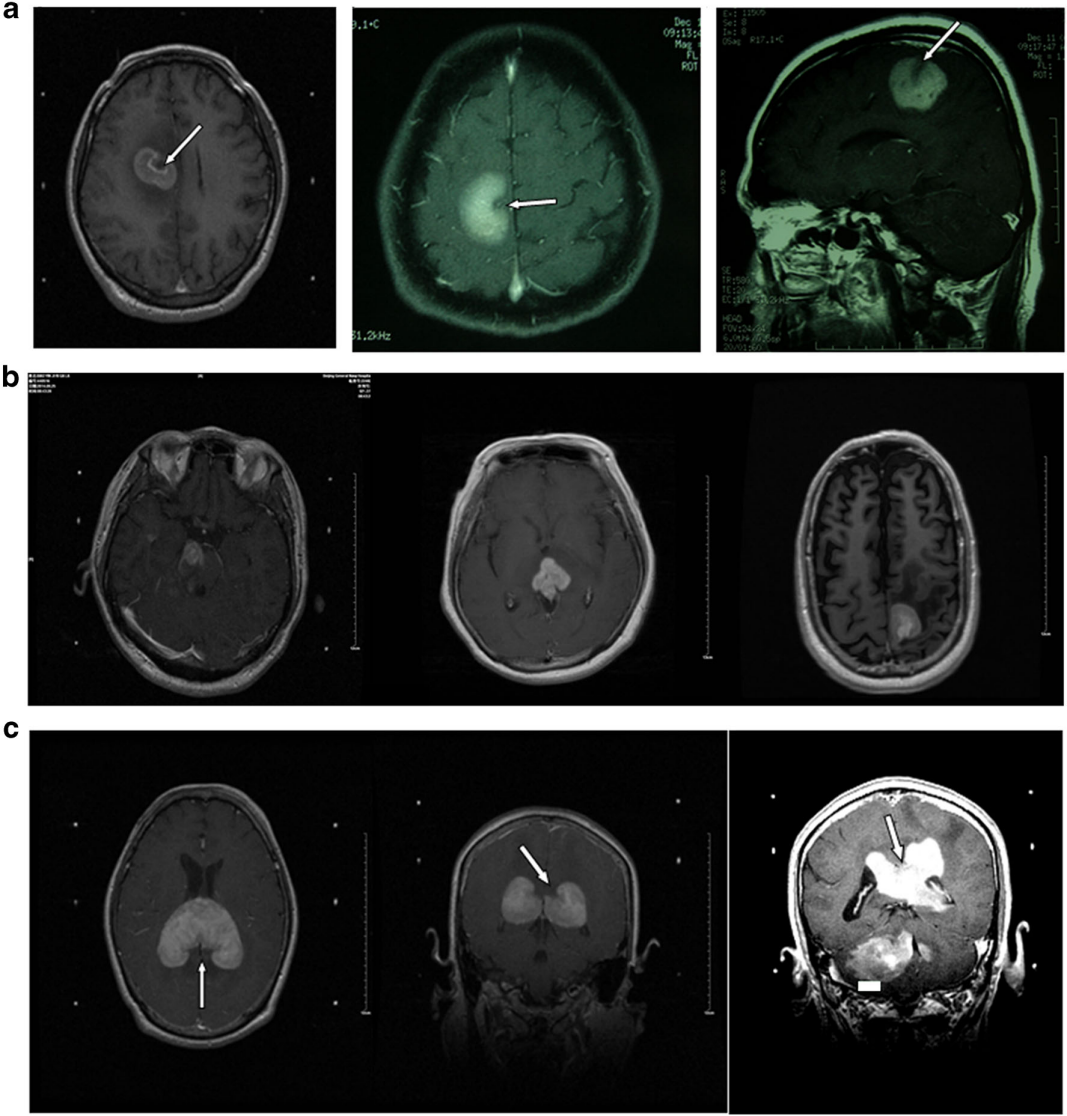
a The typical “belly button” sign of primary central nervous system lymphoma (PCNSL) on magnetic resonance imaging (MRI) (arrow). b The “snow ball” sign of primary central nervous system lymphoma (PCNSL) on MRI. c The “butterfly sign” of primary central nervous system lymphoma (PCNSL) on MRI
